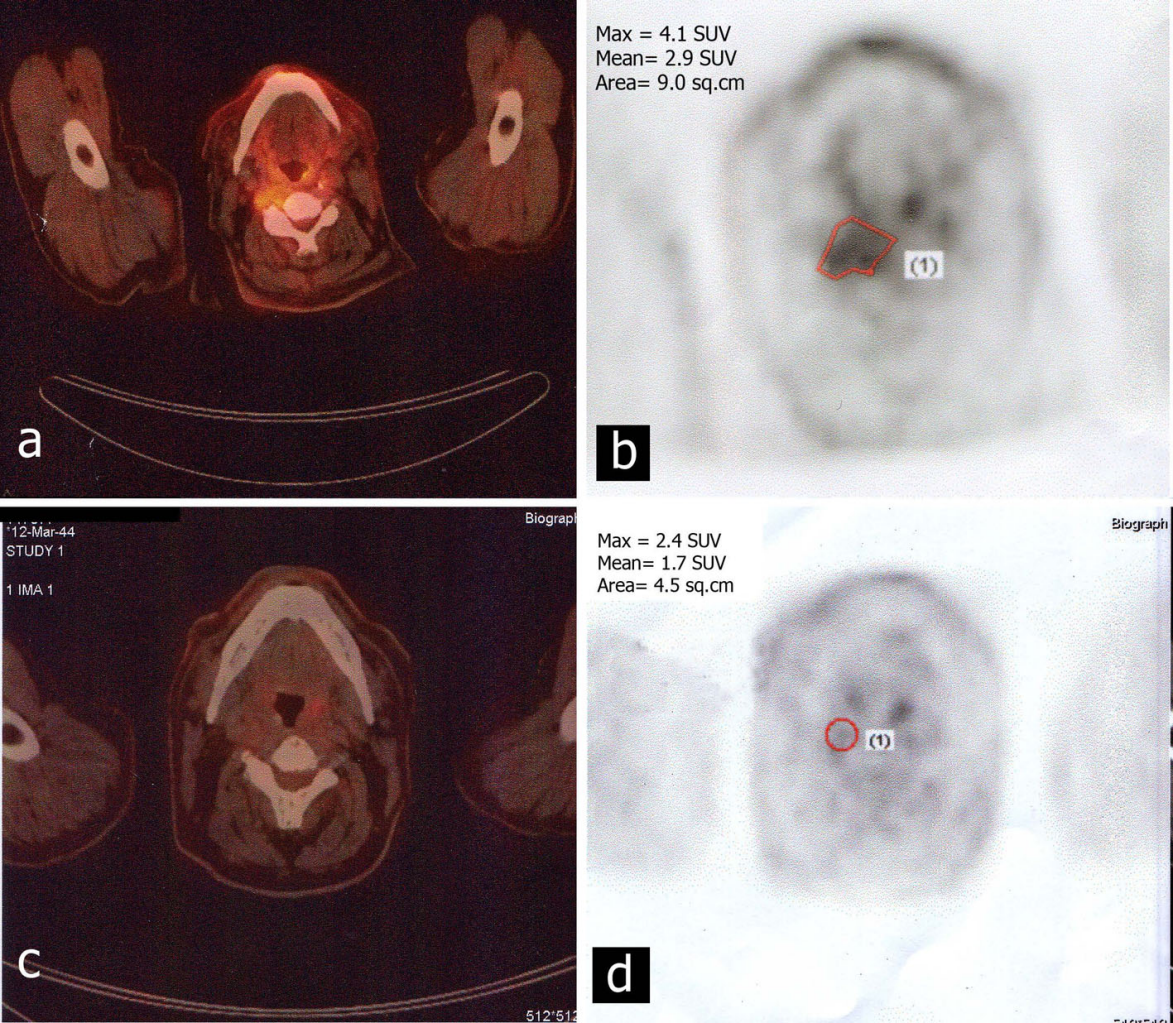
Metabolic response of one patient treated with imatinib mesylate. Comparison of FDG PET imaging pre- (a, b) and post- (c, d) three months of therapy.
